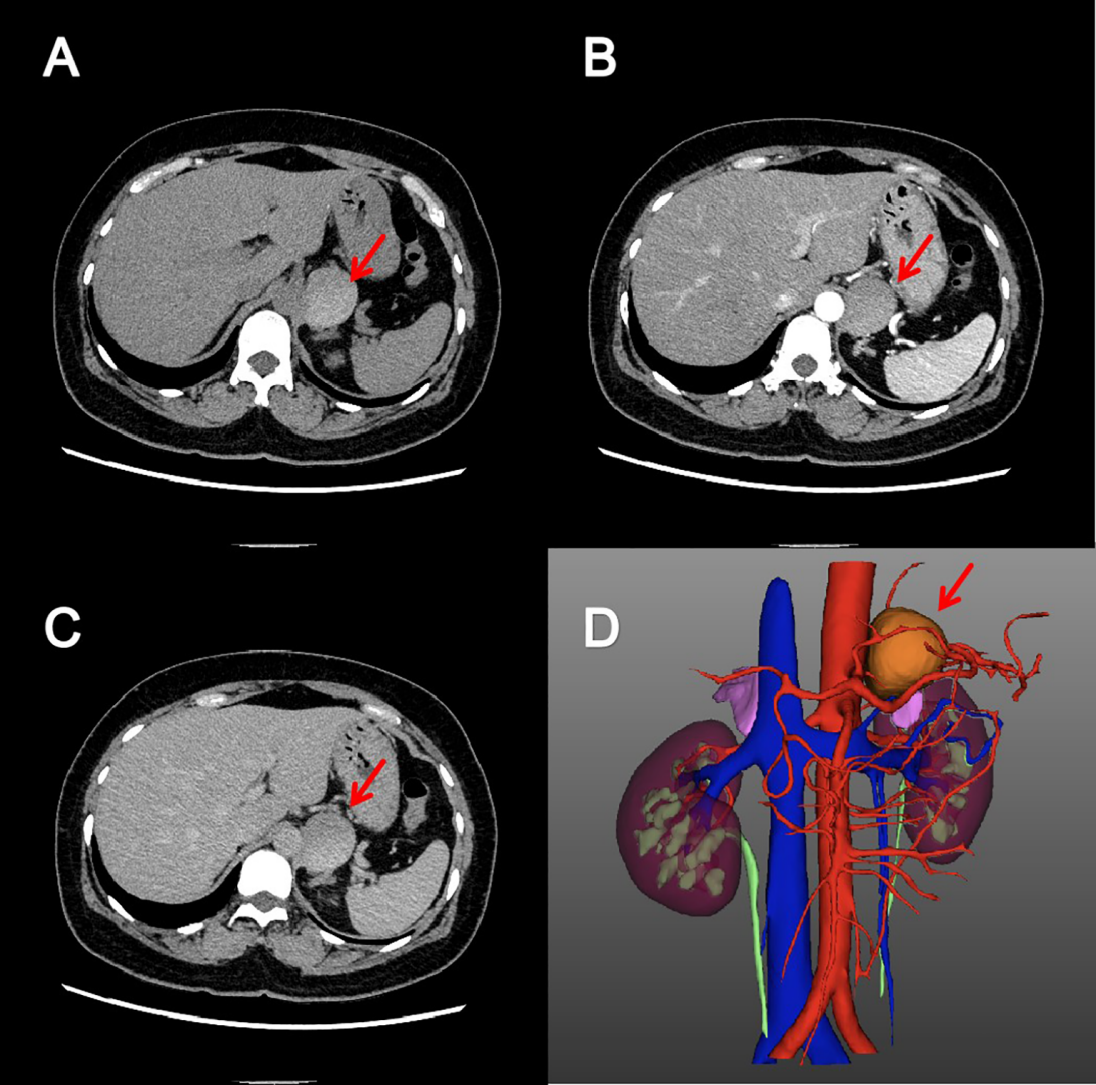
Adrenal enhanced computed tomography image. Axial pre-contrast (A), arterial phase (B) and excretory phase (C) CT images showed a cystic, well-circumscribed mass without enhancement in the left adrenal region. The reconstructed three-dimensional image (D) showed that the mass was closely related to the left adrenal gland.
